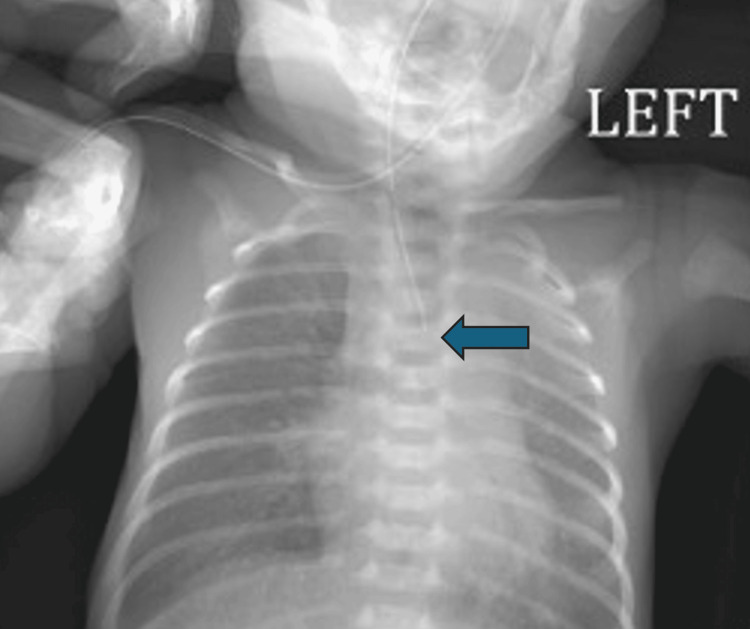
Chest x-ray demonstrating NG tube coiled at the level of T4 concerning for esophageal atresia without fistula. NG: nasogastric
